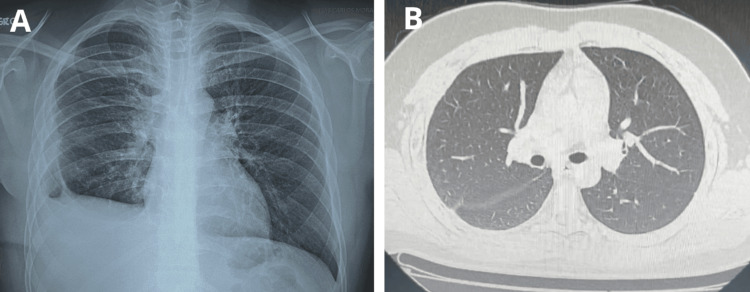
Chest X-ray showing adequate lung re-expansion and elevation of right hemidiaphragm (A). CT image in the lung window with resolution of empyema with good response and lung re-expansion (B).
